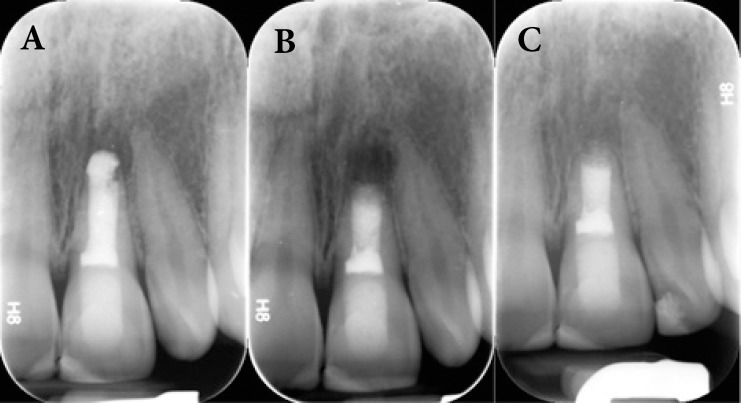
A) Preoperative periapical radiograph of endodontically treated right central incisor; B) Periapical radiograph after CEM cement root-end filling; C) Periapical radiograph after one year follow-up shows complete bone healing and establishment of periodontal ligament (PDL
